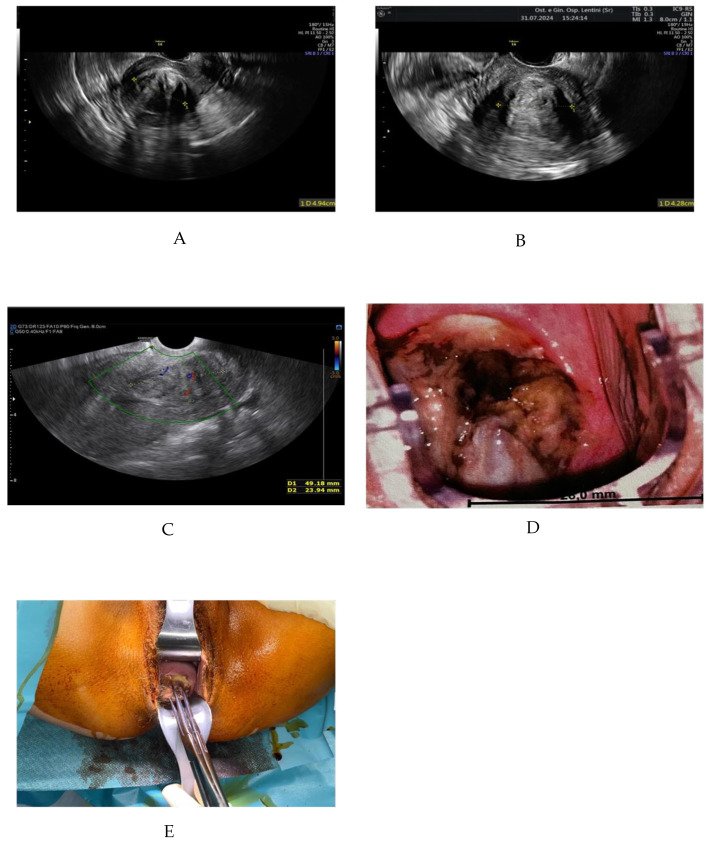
(A) Transvaginal ultrasound showing a 5 cm FIGO type 2–5 fibroid before RFA; (B) One month post-RFA, showing a reduction in fibroid size; (C) Two months post-RFA, Doppler ultra- sound revealing fibroid descent into the cervical canal; (D) Cervical fibroid visualized during colposcopic examination (28.0 mm); (E) Intraoperative image showing the removal of the expelled fibroid.
